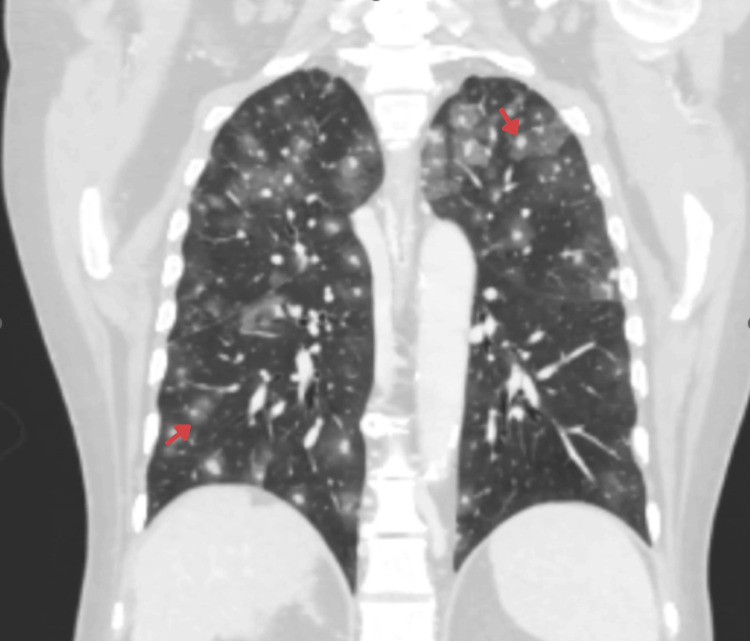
Coronal CT of the thorax showing numerous bilateral pulmonary nodules. Many nodules are surrounded by a peripheral ground-glass halo, the “halo sign,” indicating perilesional hemorrhage which is highly suggestive of hemorrhagic metastases from angiosarcoma.
